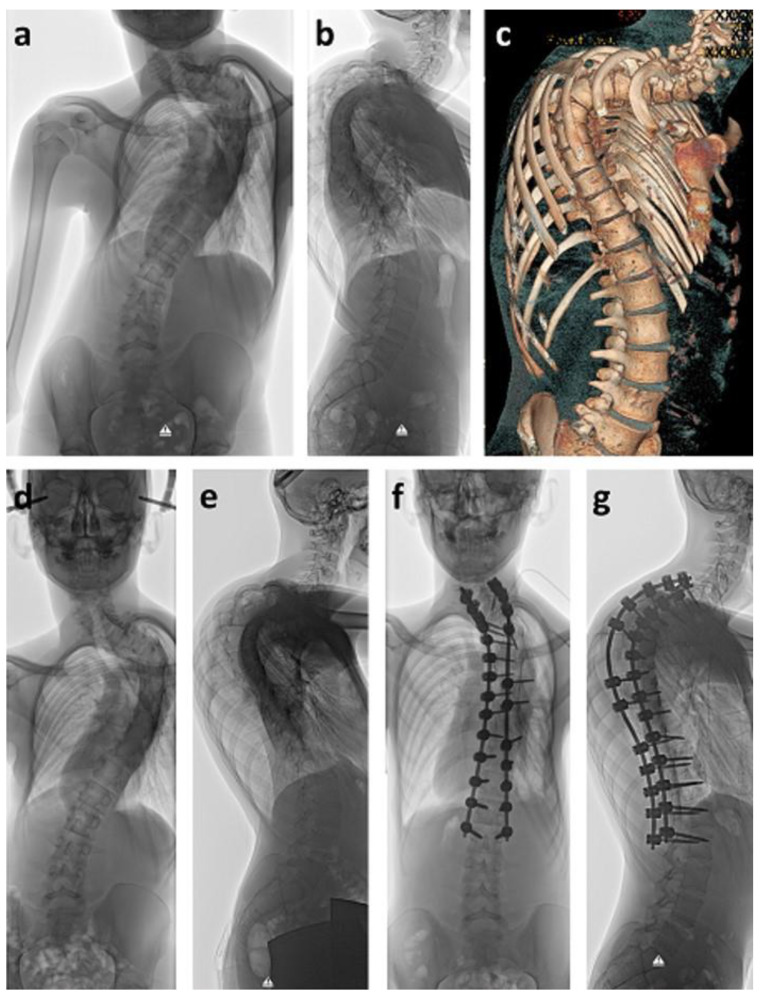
Fifteen-year old boy who was diagnosed with intraspinal astrocytoma at age ten. He received chemotherapy and radiotherapy. Five years later, severe thoracic spinal deformity had developed (a–c). Halo traction was applied for gradual scoliosis and kyphosis correction as well as reduction of the surgical risk due to neurological deterioration (d,e). Surgery was performed using spinal cord monitoring. When erecting the spine, motor evoked potentials (MEP) disappeared. After controlling correct screw placement by computed tomography, spinal deformity correction could only be performed until MEP weakened, thus leaving considerate kyphosis as a residual deformity (f,g).
